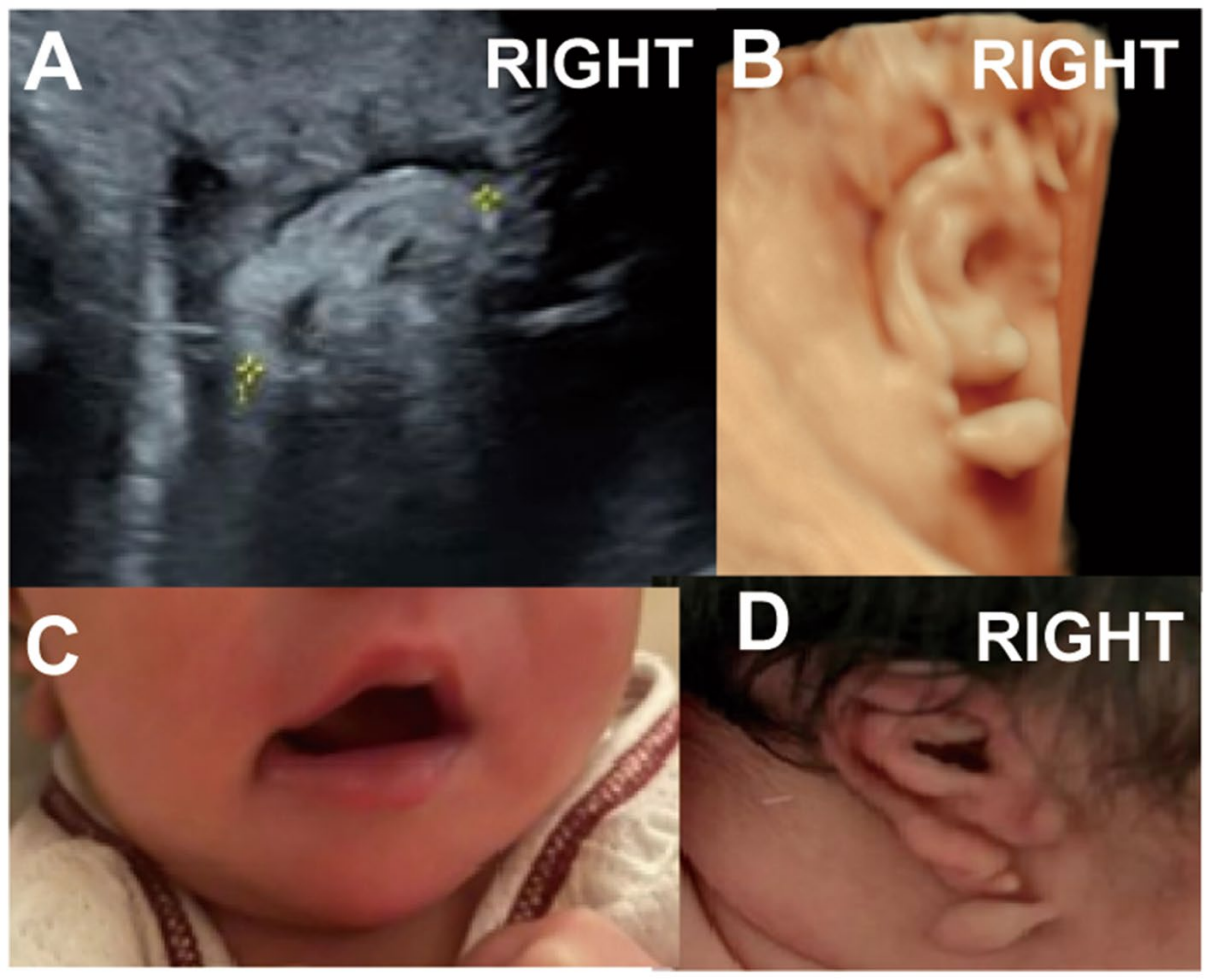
(Case 14). At 24 weeks’ gestation, ultrasound indicated a right ear deformity, prompting a referral. (A,B) By 28 weeks, the left ear measured 2.6 cm with normal morphology, while the right ear measured 2.1 cm and showed abnormal structure with a lump beneath the earlobe. (C,D) A full-term baby girl was delivered with a question mark ear deformity and a lateral facial cleft on the right side. Hearing tests showed elevated ABR response thresholds, delayed wave latencies, and a possibly prolonged T-V interval on the right side.
